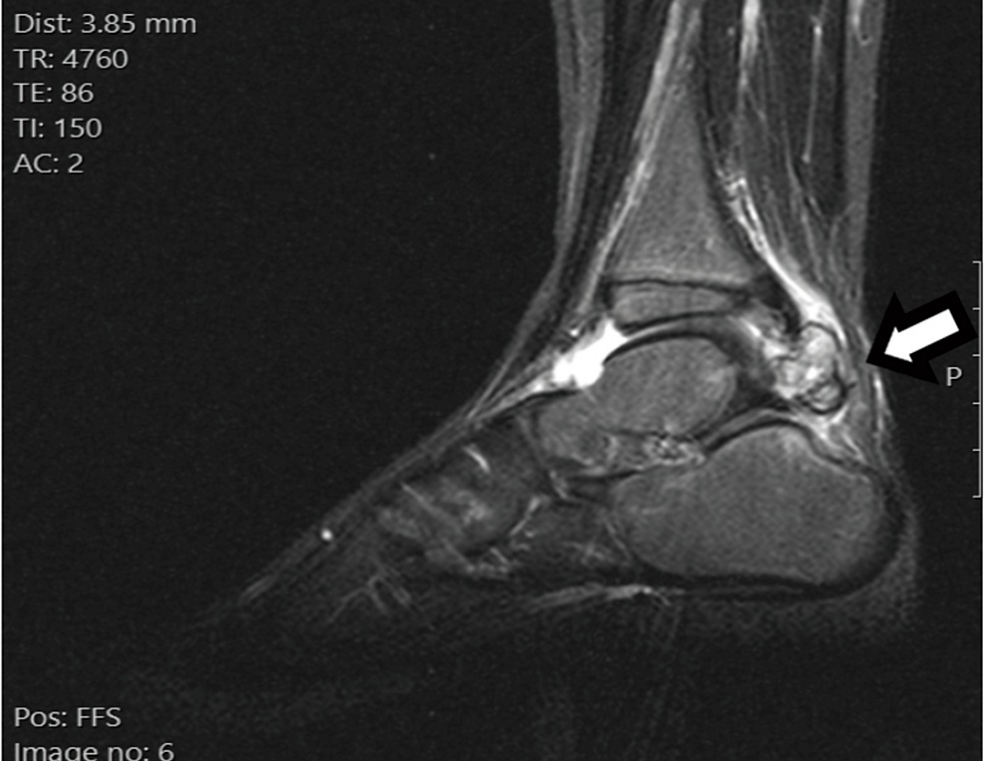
MRI scan of the right ankle sagittal STIR sequence. Short tau inversion recovery (STIR) sequence in the sagittal plane demonstrates a large lobulated mass in the posterior aspect of the ankle joint, which projects in the Kager’s fat pad. The mass has an inhomogeneous appearance, with interchanging areas of high and low magnetic resonance signals. The lesion is in close proximity to the cortex of the posterior surface of the talus, without clear evidence of a bony protuberance that connects the lesion with the host bone. An increased amount of fluid is observed in the ankle joint.
